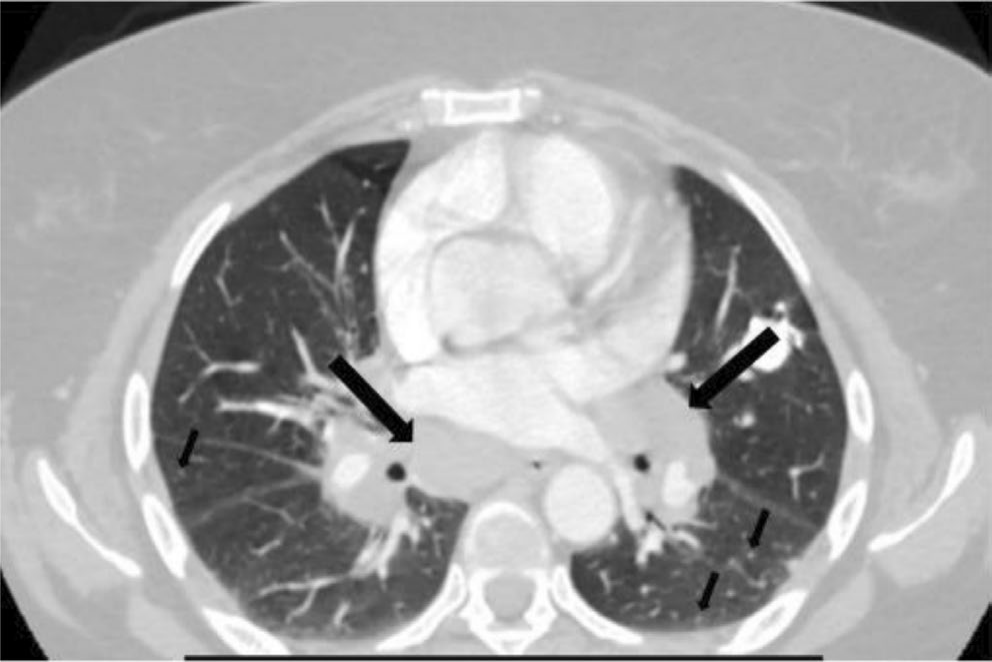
Bilateral hilar lymphadenopathy (big arrows) and multiple perilymphatic nodules (small arrows) on chest CT scan.
